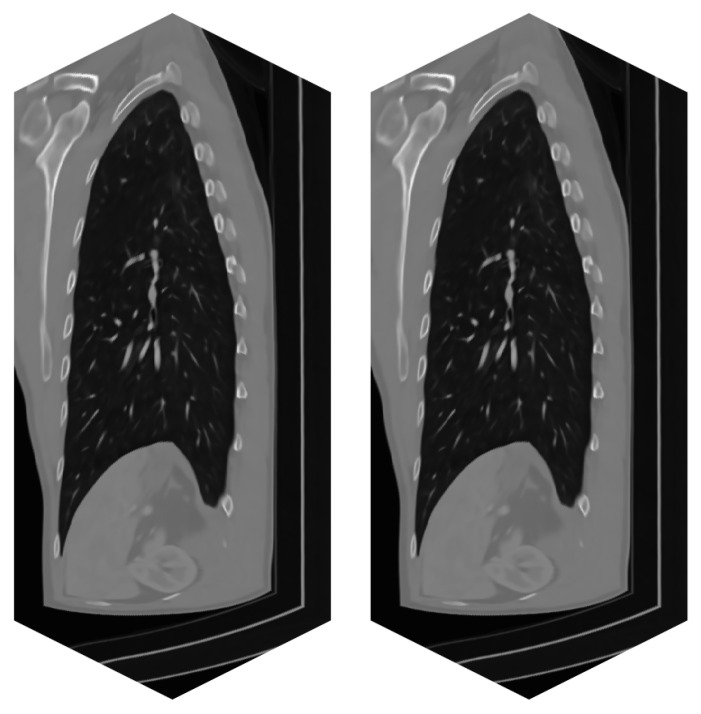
Real CT data of a patient—series of 779 images. (left)—Original noisy data. (right)—Filtered image data by BM4D.
